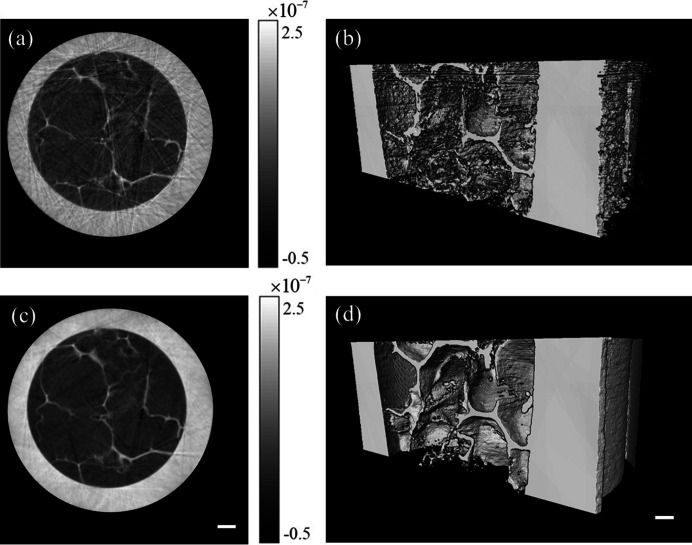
CT images of the artificial sponge reconstructed with the real part of the refractive index: (*a*) slice reconstructed by the traditional speckle-tracking method; (*b*) longitudinal section of the corresponding 3D image; (*c*) and (*d*) results of the deep-learning based method corresponding to the slice and 3D image, respectively. The scale bar represents 500 µm.
